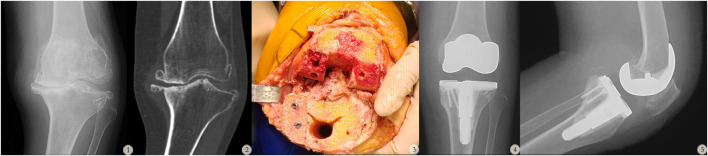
A 59-year-old female with Rand II type bone defect in the left knee. (1–2: Preoperative X-ray and CT scan of the patient’s left knee. 3: Intraoperative images showing the use of a tibial stem extender combined with two cancellous screws to address the bone defect during TKA surgery. 4–5: Postoperative follow-up X-rays of the patient’s left knee.)
